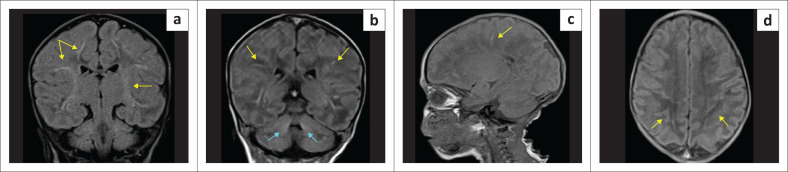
(a-d) Day-17-neonate with seizures because of neonatal *gram-negative meningo-encephalitis*. Note an unusual presentation with FLAIR and T1-W hyperintensity in the white matter (yellow arrows) extending along some perivascular spaces due to exudate. There is also mild signal change around the fourth ventricle and dentate nuclei (cyan arrows).
